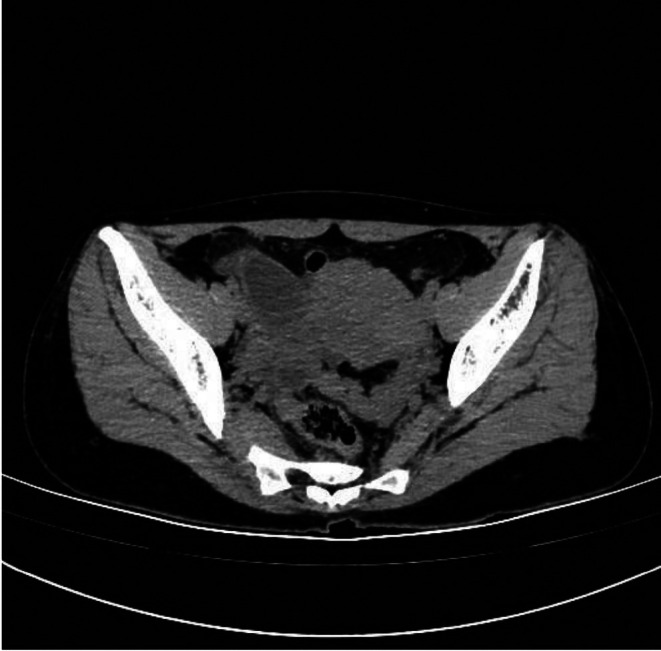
Computed tomography showed cystic low‐density lesions in the area of the right lower abdomen.
